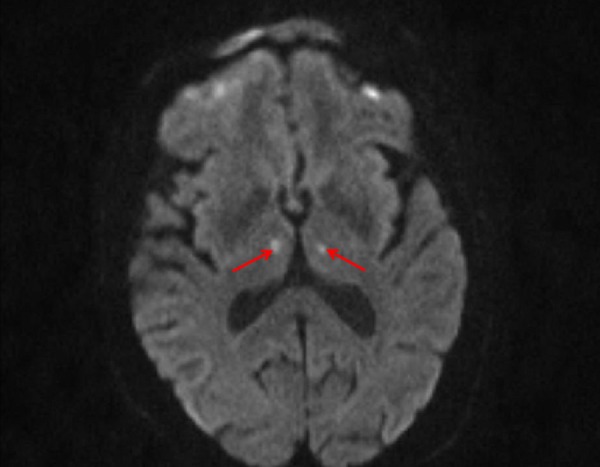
Magnetic resonance imaging (MRI) demonstrating bilateral medial thalamic infarcts Axial diffusion-weighted imaging (DWI) sequence showing restriction of diffusion in both medial thalami (red arrows)
